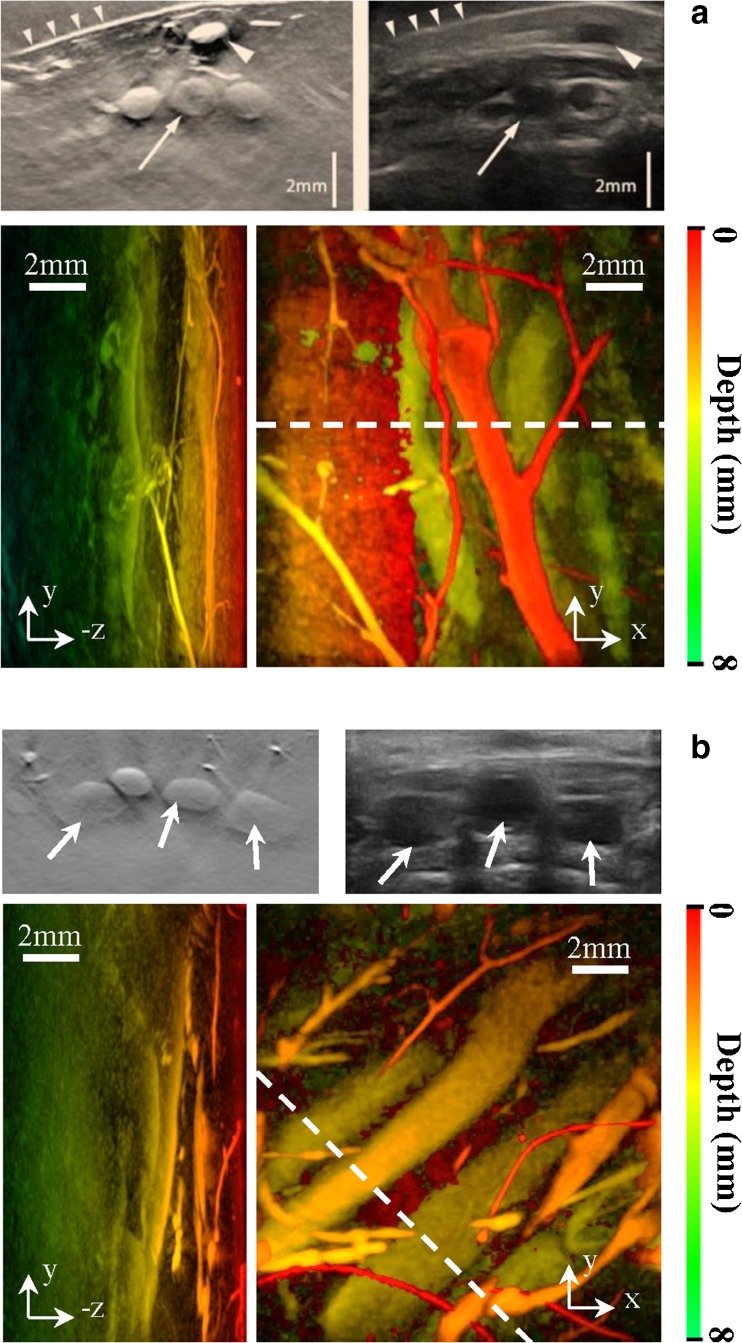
Example short axis 2D slices taken from the volumetric PA imaging series (*left upper panels*) with contemporaneous, location-matched ultrasound (*right upper panels*) for two separate volunteers (a and b respectively). The corresponding PAI volumetric data sets, presented as coronal and sagittal maximum intensity projection (MIP) images, are shown in the *lower panels*. MIP images are color-coded for depth (see scale). The *dashed line* indicates the plane through which the axial image has been reconstructed. The DP artery, with its accompanying venae comitantes, is arrowed, with a superficial vein (*large arrowhead)* and the skin surface (*small arrowheads*) also shown in a. The axes are as follows; y, proximal-distal; x, medial-lateral; z, dorsal-plantar
